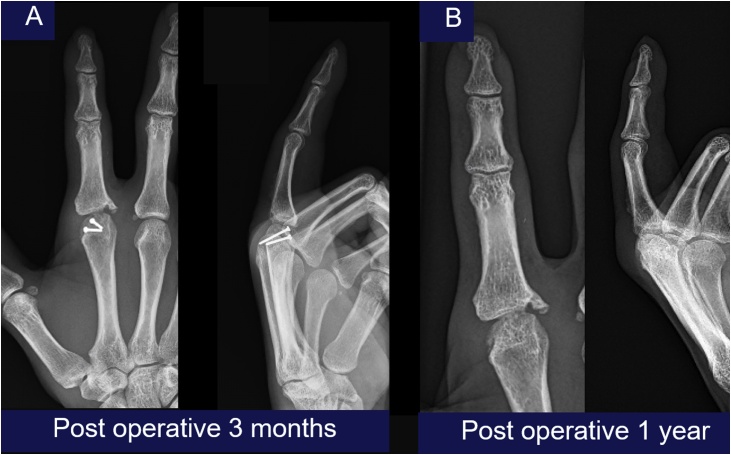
The radiographs showed the union of intraarticular fracture at 3 month follow up (Fig. 4A) and 1 year follow up (Fig. 4B).
